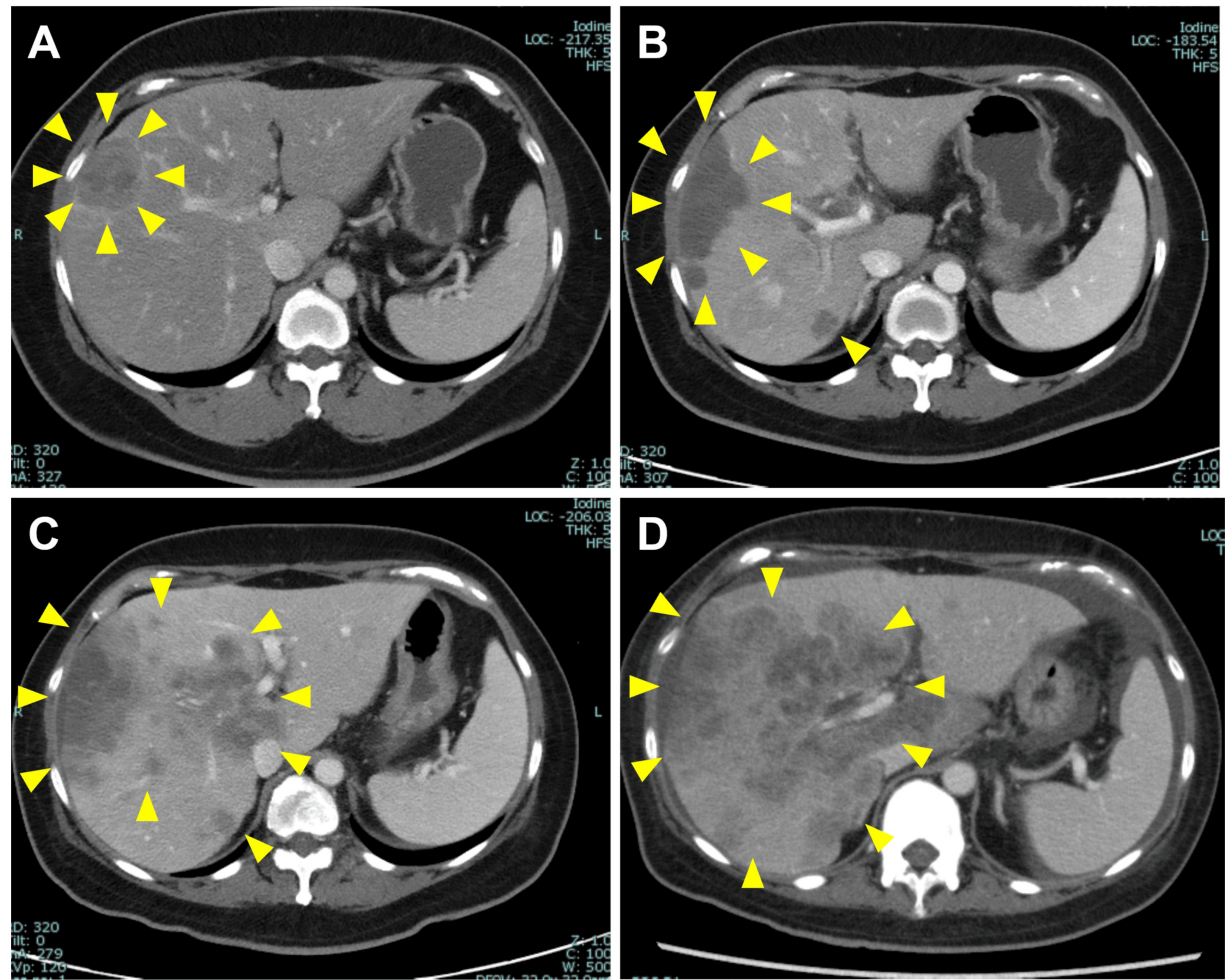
Computed tomography images of liver metastases (A) Twenty months after the primary treatment, computed tomography revealed multiple liver metastases. Computed tomography scans of the liver metastases were taken after (B) five cycles of lenvatinib plus pembrolizumab, (C) four cycles of doxorubicin and cisplatin, and (D) two cycles of paclitaxel and carboplatin. Yellow arrowheads indicate liver metastases.
